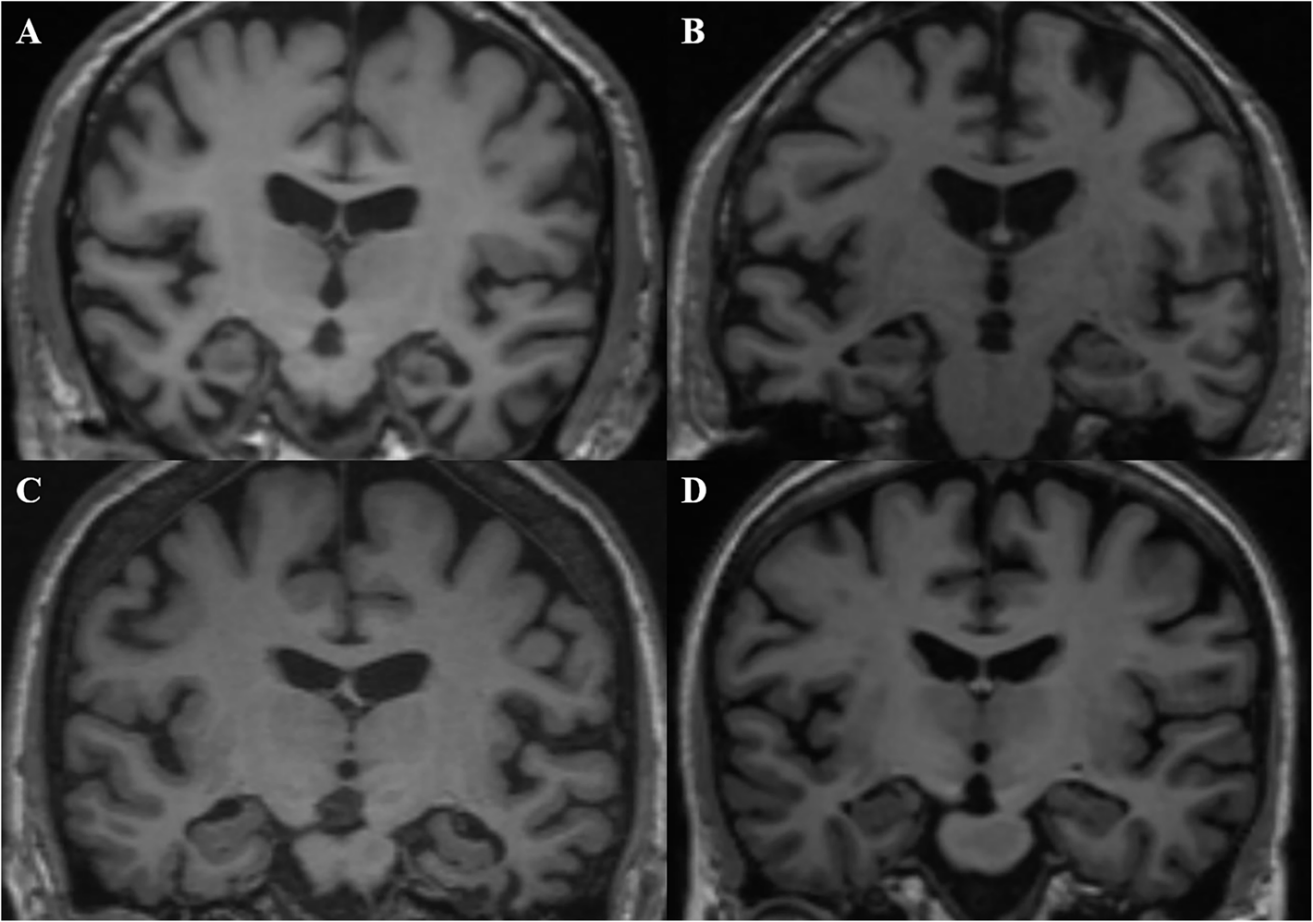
3D T1-weighted MRI scans (classical coronal section) of a FTD with CDR = 0.5, ID NACC204983; b AD with CDR = 0.5, ID NACC162576; c FTD with CDR = 1.0, ID NACC067187; and d AD with CDR = 1.0, ID NACC878860
